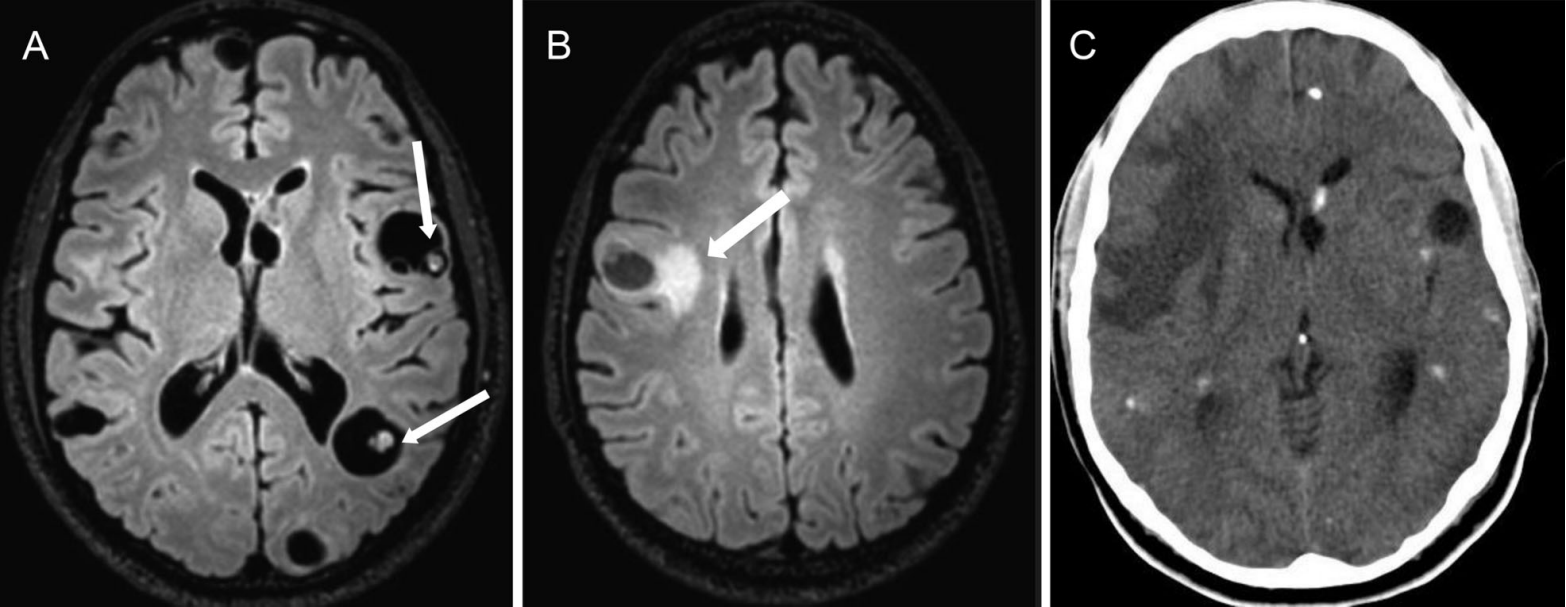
Disseminated parenchymal neurocysticercosis in a 23-year-old man with intractable epilepsy and multiple epileptic foci (video-EEG). A) An axial T2 FLAIR sequence showing multiple vesicular cysts, some with scolex inside (white arrows). B) An axial T2 FLAIR image revealing perilesional edema in the right frontal lobe (white arrow). C) An axial TC shows multiple calcified lesions. These findings characterize the different phases of neurocysticercosis, which are pathognomonic of this disease.
